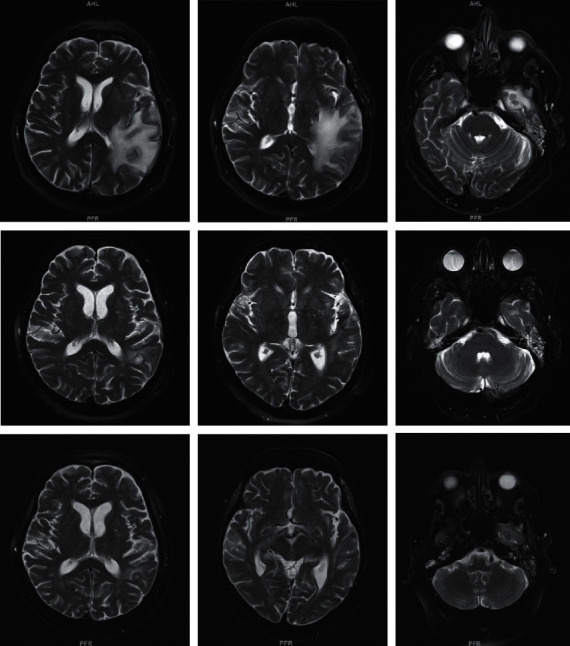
T2-weighted sequences of the brain MRI demonstrating cerebral abscesses and associated edema. Top row: initial presentation; middle row: repeat imaging, 3 weeks after initial presentation; bottom row: final imaging, 2 months after initial presentation.
